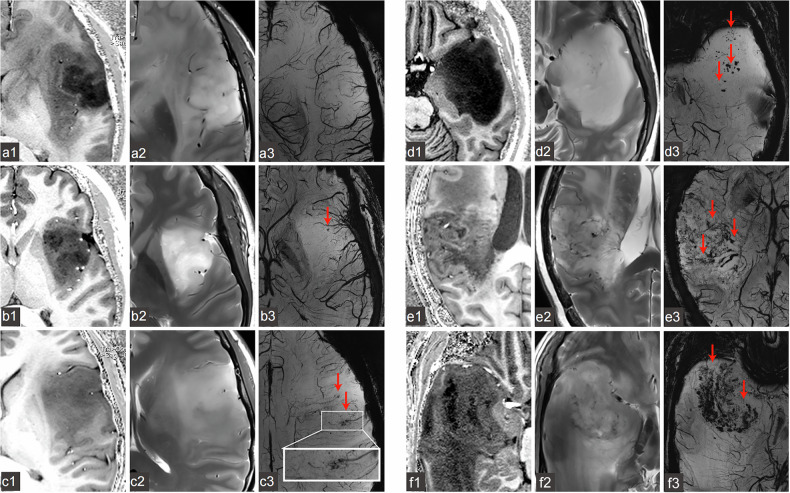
Intratumoral susceptibility signals (ITSS) in different grades of glioma (indicated by arrows). Demonstrated through three sequences: T1-weighted imaging (a1, b1, c1, d1, e1, f1), T2-weighted imaging (a2, b2, c2, d2, e2, f2), and SWI (a3, b3, c3, d3, e3, f3). a1–a3 Astrocytoma WHO II, ITSS 0 (ITSS = 0); b1–b3 Oligodendroglioma WHO II, ITSS 1 (1 ≤ ITSS ≤ 10); c1–c3 Astrocytoma WHO III, ITSS 2 (11 ≤ ITSS ≤ 35); d1–d3 Glioblastoma WHO IV, ITSS 3 (36 ≤ ITSS ≤ 50); e1–e3 Glioblastoma WHO IV, ITSS 4 (51 ≤ ITSS ≤ 85); f1–f3 Glioblastoma WHO IV, ITSS 5 (ITSS > 85)
